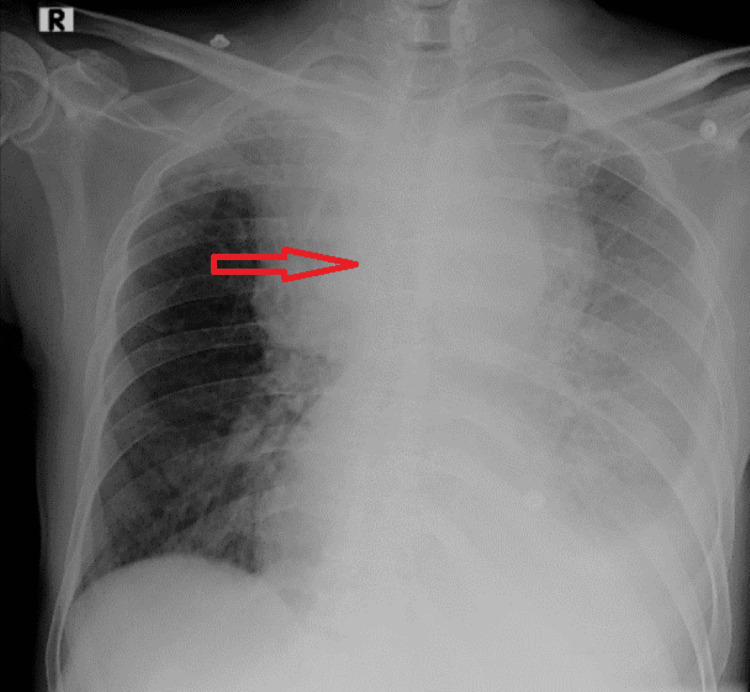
Chest X-ray showing widened mediastinum and left sided pleural effusion.
